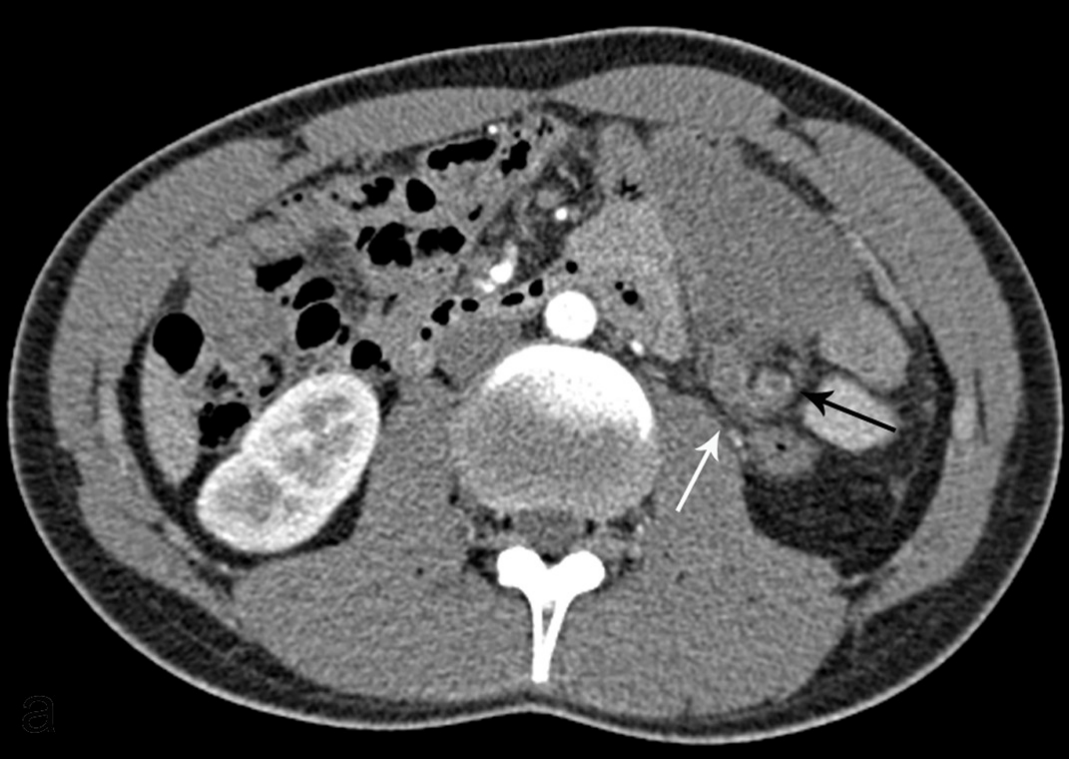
Axial CT arterial phase image shows hypoattenuating left flank mass corresponding to the wandering spleen. The splenic pedicle is twisted (white arrow), giving a whirled appearance that confirms the diagnosis of torsion. The central structure of the whirl is the splenic artery with thrombus inside (black arrow), and the peripheral part corresponds to the vein with thrombus (confirmed in venous phase images, not shown).
